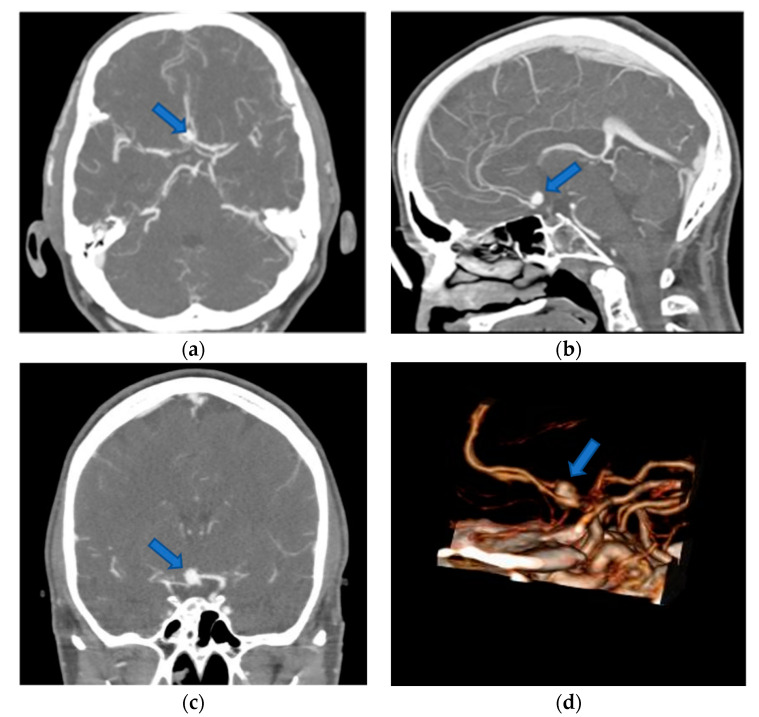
Preoperative 2D-CTA and screen 3D-CTA images of patients with unruptured ACoA aneurysm (blue arrows). (a) Axial 2D-CTA image presenting anteriorly directed ACoA aneurysm; (b) sagittal 2D-CTA image presenting superiorly directed ACoA aneurysm; (c) coronal 2D-CTA image presenting superiorly directed ACoA aneurysm; (d) lateral screen 3D-CTA image presenting anteriorly directed ACoA aneurysm. 2D, two-dimensional; 3D, three-dimensional; ACoA, anterior communicating artery; CTA, computed tomography angiography.
